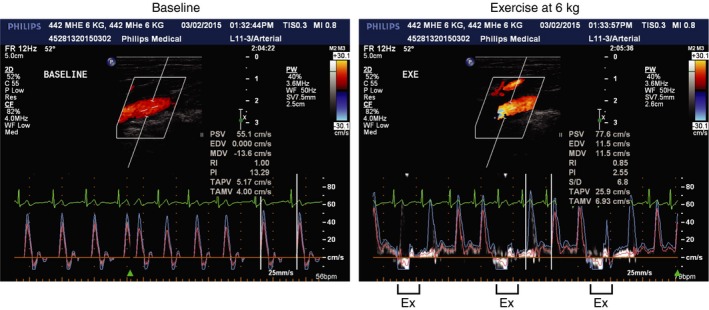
Original Doppler ultrasound recordings of popliteal blood flow velocity recorded at baseline (left panel) and during single‐leg dynamic plantar flexion exercise at 6 kg (right panel) in a 62‐year‐old healthy man. Note that in the left panel, velocity waveforms are triphasic whereas in the right panel the velocity waveforms are not triphasic and can only be measured in between exercise (Ex) contractions.
